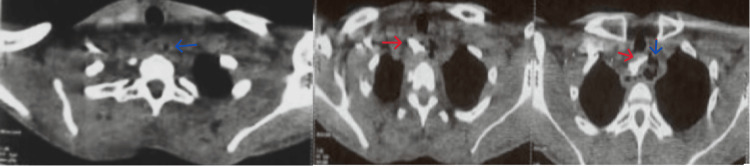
Cervicothoracic CT scan Axial cervicothoracic CT scan showing mediastinal air and fat infiltration( blue arrow), foreign body (red arrow)
